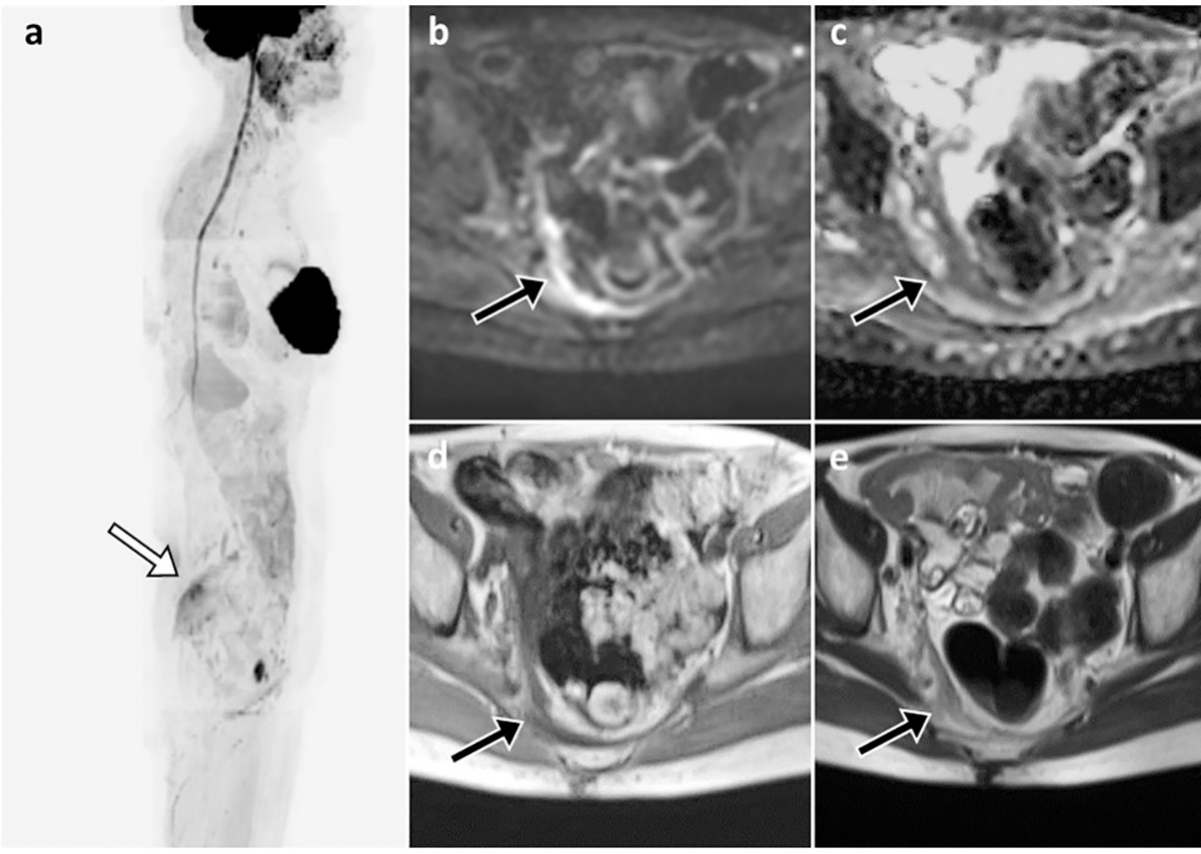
In the same patient of Fig 5, the paired WB-MRI showed thickening of the peritoneum in the right pelvis, which was hyper-intense in DWI b-900 sagittal MIP (a) and DWI axial b-900 images (arrow in b), with corresponding reduced ADC values (c). Axial T1 and T2 images confirmed the presence of a suspicious thickening of the right pelvic peritoneum and mesorectal fascia (arrows in d and e). No significant fluid collection can be seen. Other benign high signal intensity areas in the MIP image (a) include salivary glands, spinal cord, silicon breast implant, spleen, kidneys, small bowel and a bartholin’s gland cyst. The PD reported at WB-MRI determined a change in treatment from Letrozole to Fulvestrant.
